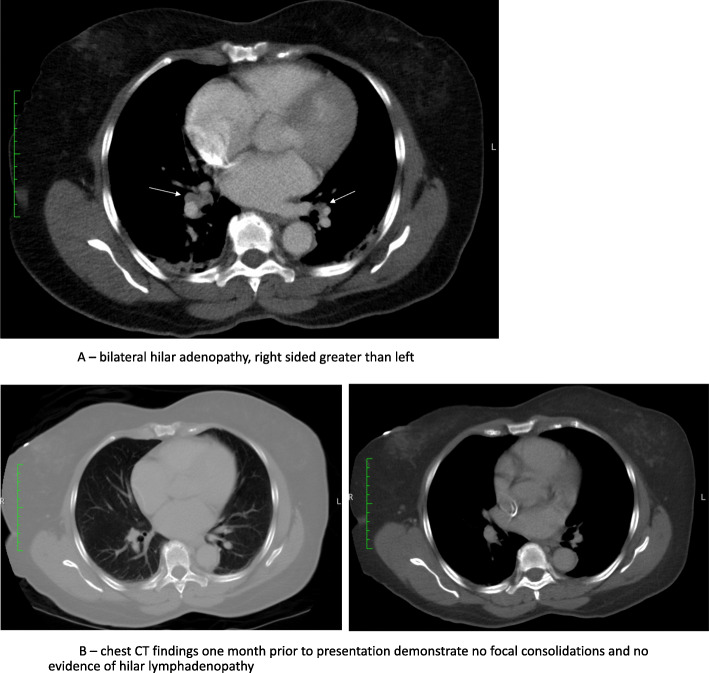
a Atypical bilateral hilar lymphadenopathy (arrow), a novel finding of coronavirus disease 2019. b Chest computed tomography 1 month before presentation demonstrates no focal consolidations and no lymphadenopathy
